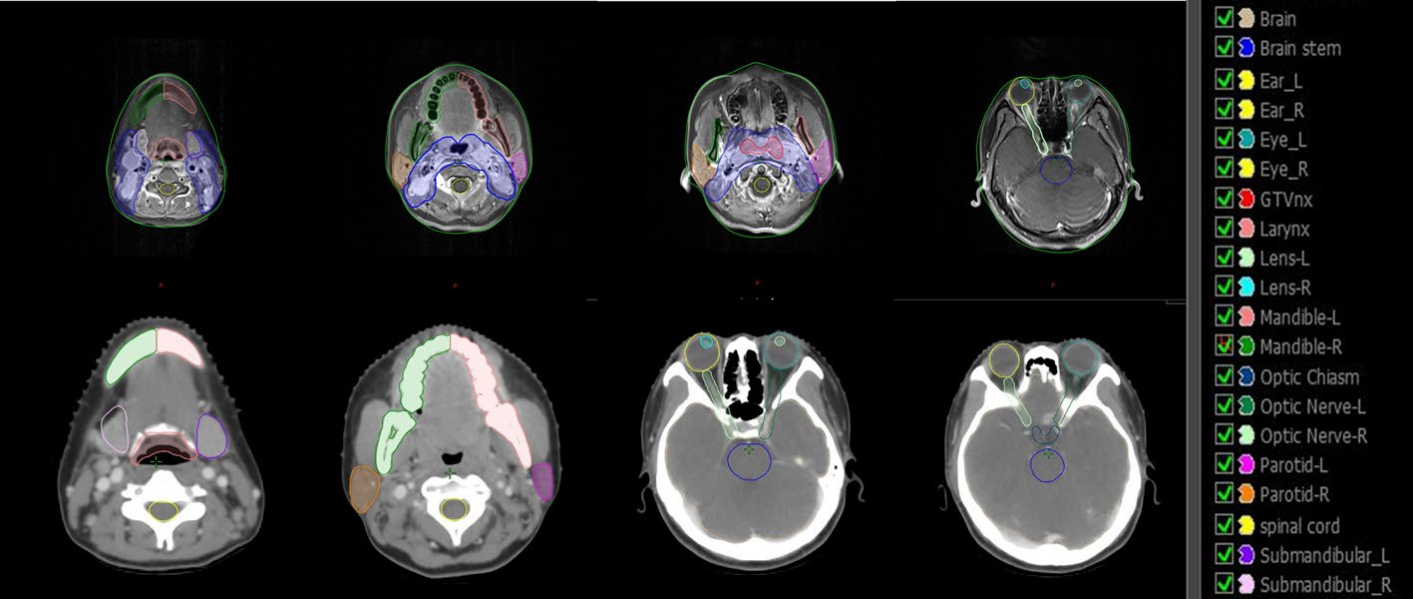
Sample CT/MRI image slices with OARs contours
